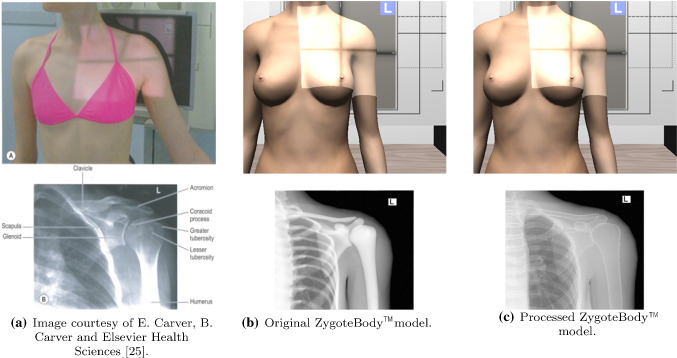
Proposed system can replicate any type of projection, allowing the user to select the patient position and the X-ray configuration
